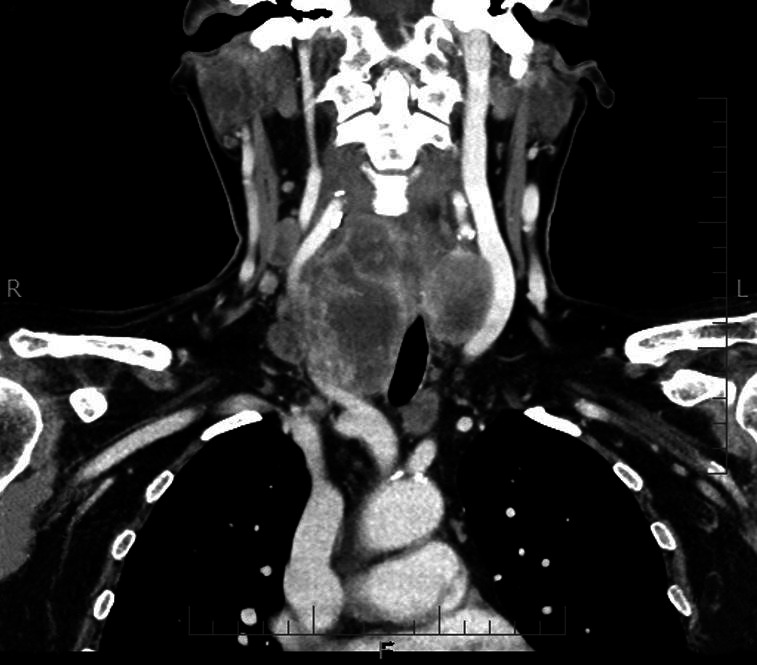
Cervical computed tomography scan showing voluminous tumor of the thyroid gland with a preponderating mass in the right lobe embedding the right carotid artery.
